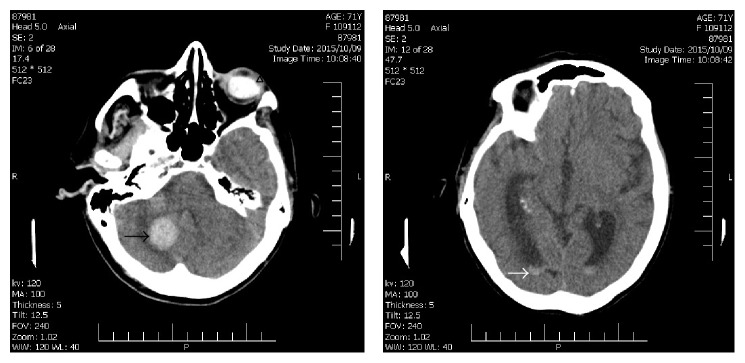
Brain CT scan. Hyperdensity in vitreous cavity of the left eye (arrowhead), hemorrhage in right cerebellum (black arrow), and intraventricular hemorrhage (white arrow).
